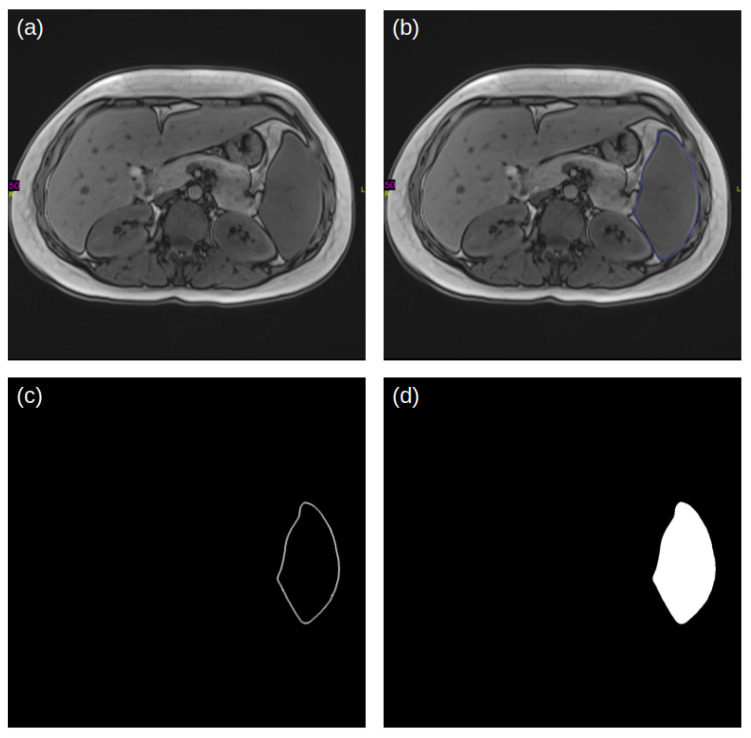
Example of an MRI slice (a), and the manual outline of the spleen in the purple color on the MRI slice (b). The pure outline of the spleen is extracted and shown in (c), and the filled outline (d) creates the mask for the spleen.
